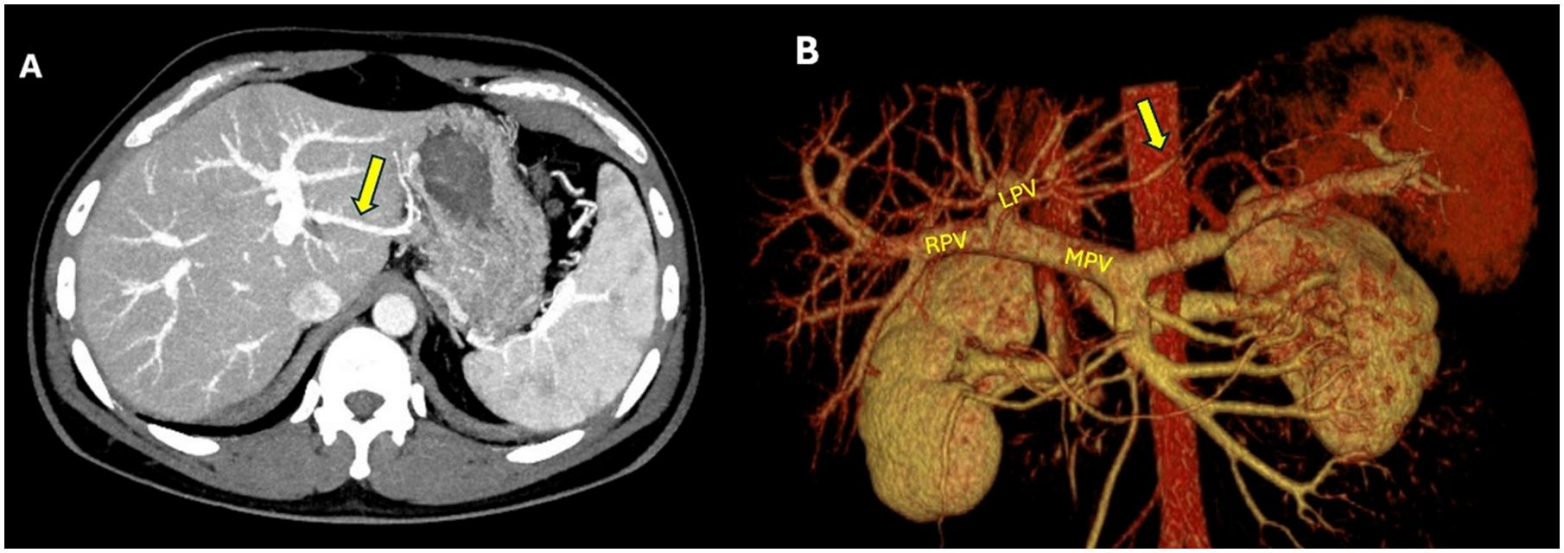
(A) Axial portal phase contrast-enhanced CT maximum intensity projection (MIP) image shows the aberrant left gastric vein draining into the left main portal vein (Type 3) (arrow). (B) 3D reconstruction image of the same patient demonstrates the aberrant left gastric vein draining into the left portal vein (arrow).
